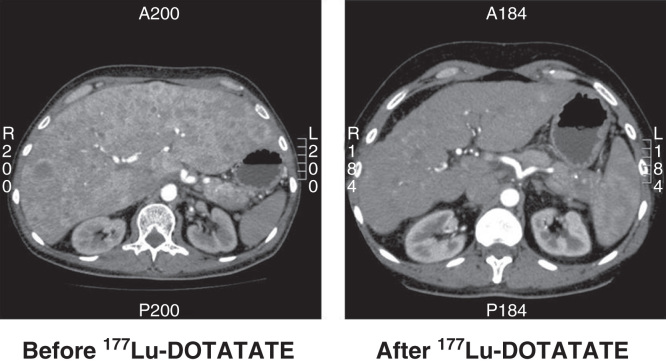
CT scan of a patient with a non-functioning pancreatic NET before and 6 months after treatment with four cycles of ^177^Lu-DOTATATE. The size of the liver is reduced and almost all metastases have disappeared.
